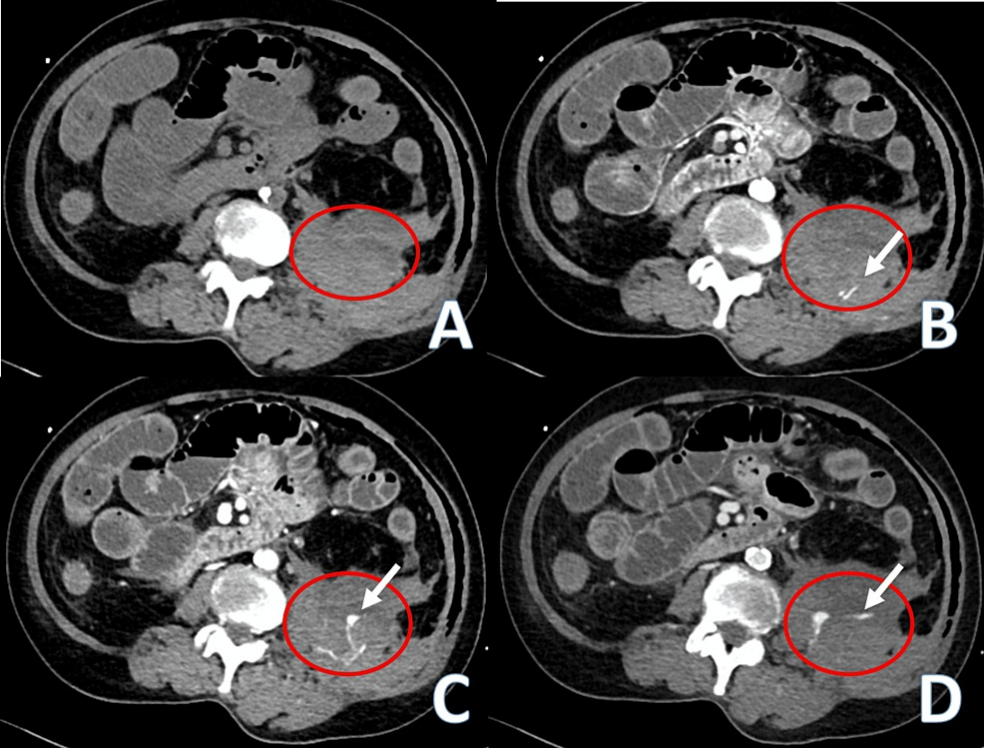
The pre-contrast (A), arterial (B), portal venous (C), and delayed (D) axial CT images showed left retroperitoneal hematoma (red circle), with active contrast extravasation (white arrow) and contrast pooling (white arrow) in the portal venous and delayed phases in keeping with active hemorrhage.
